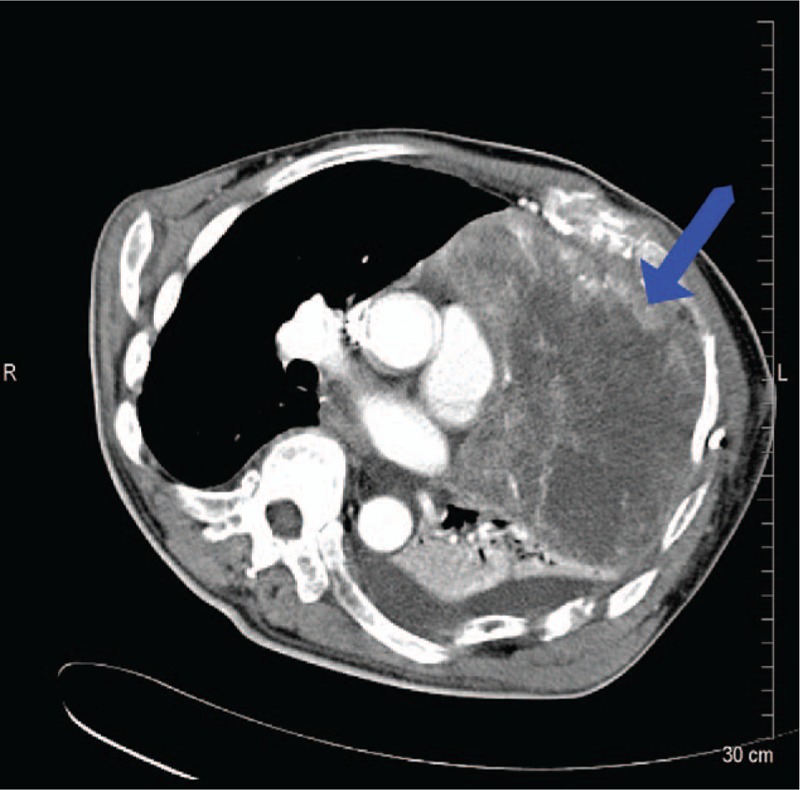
A follow-up CT of the chest at the completion of his fourth chemotherapy regimens showing tumor shrinkage between pre- and postchemotherapy (*arrow*). CT = computed tomography.
